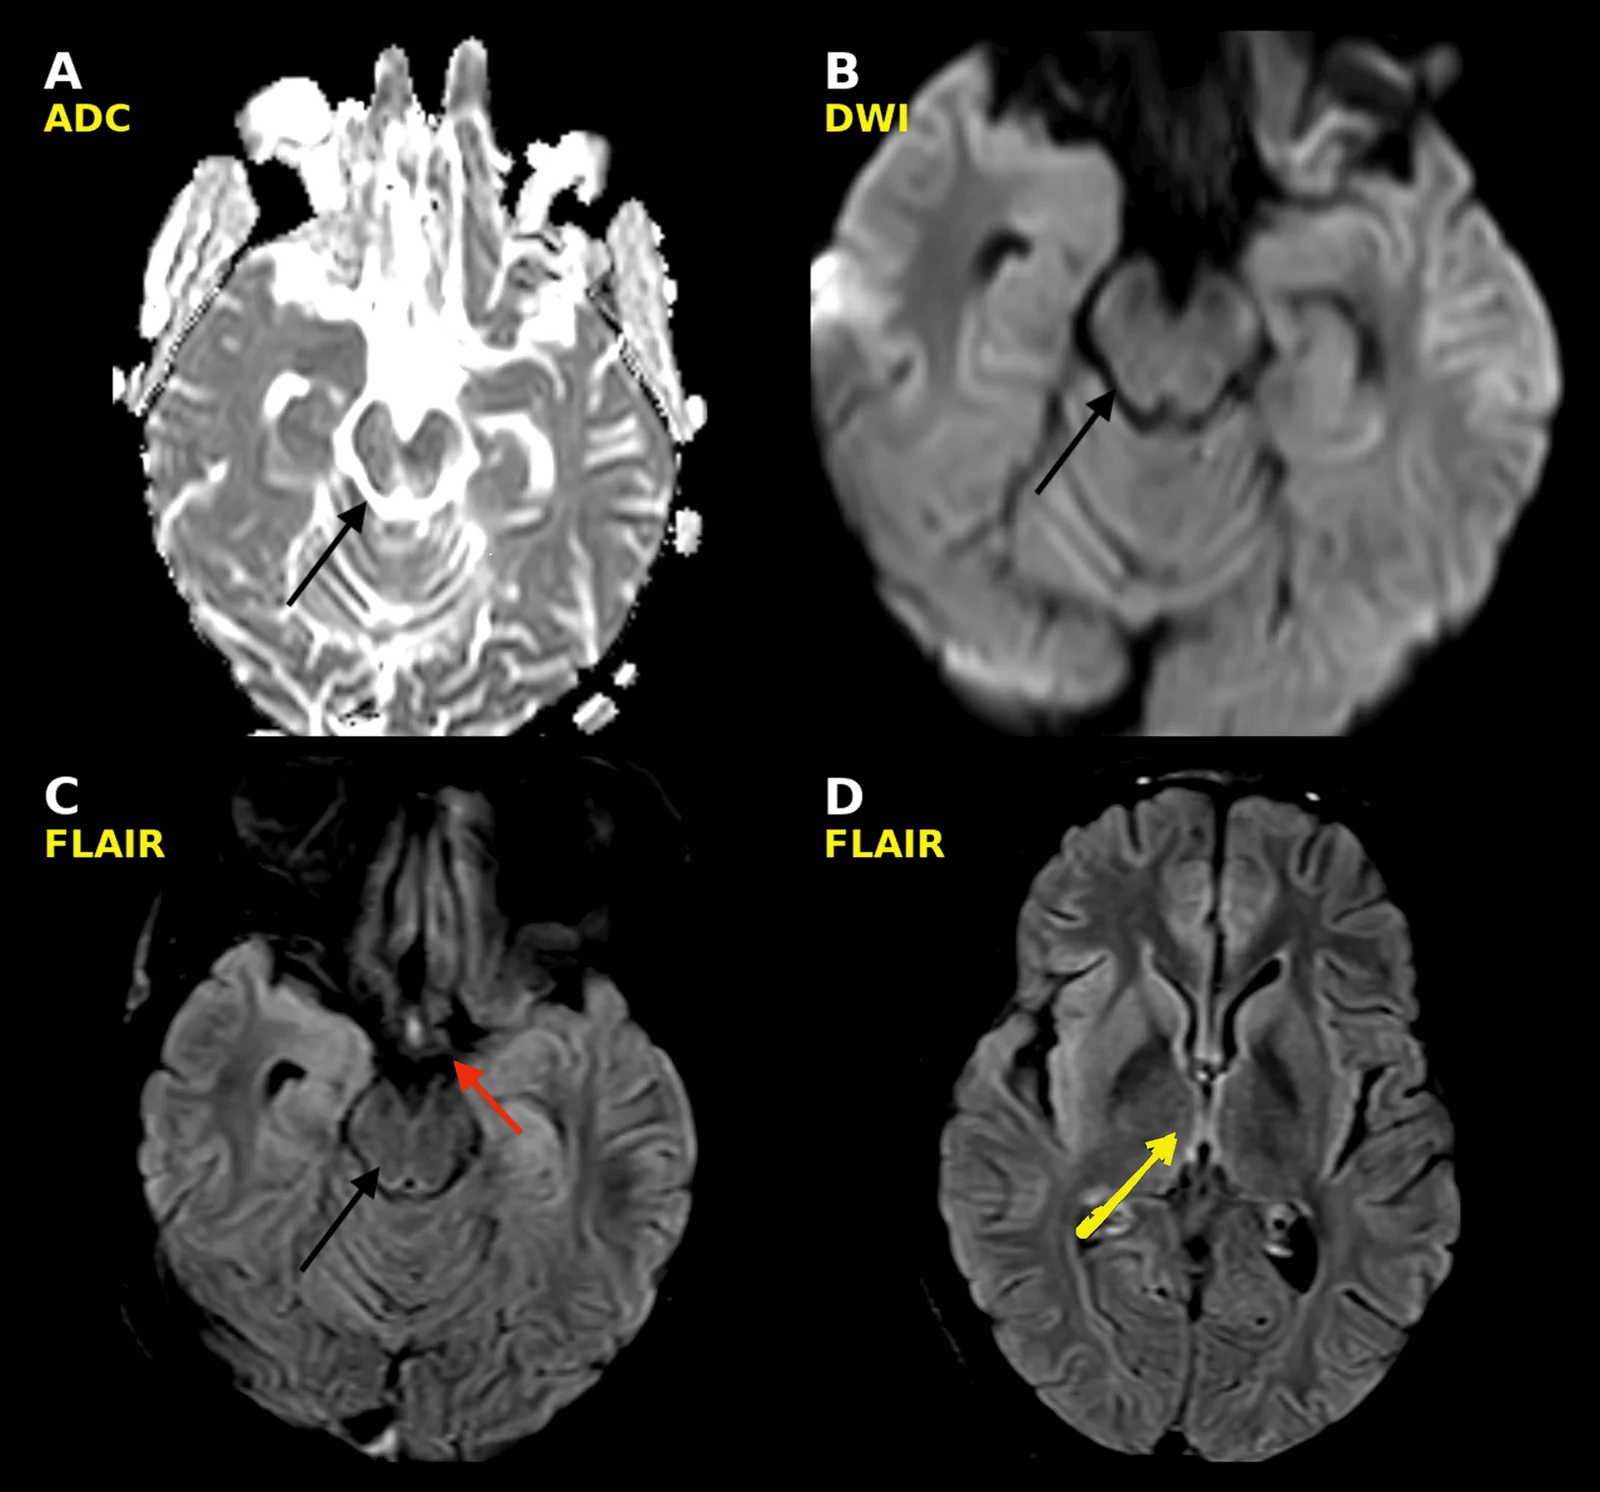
Brain magnetic resonance imaging (MRI) obtained during admission. (A) Apparent diffusion coefficient (ADC) map; the black arrow indicates the periaqueductal gray. (B) Diffusion-weighted imaging (DWI); the black arrow indicates the periaqueductal gray. (C) Axial T2 fluid-attenuated inversion recovery (FLAIR) at the level of the midbrain; the black arrow indicates the periaqueductal gray, and the red arrow indicates the mammillary body region. (D) Axial T2-FLAIR at the level of the third ventricle; the yellow arrow indicates the medial thalamus. The arrows indicate regions in which characteristic signal abnormalities may be seen in Wernicke encephalopathy (WE); no abnormal signal was identified in these regions in this patient.
